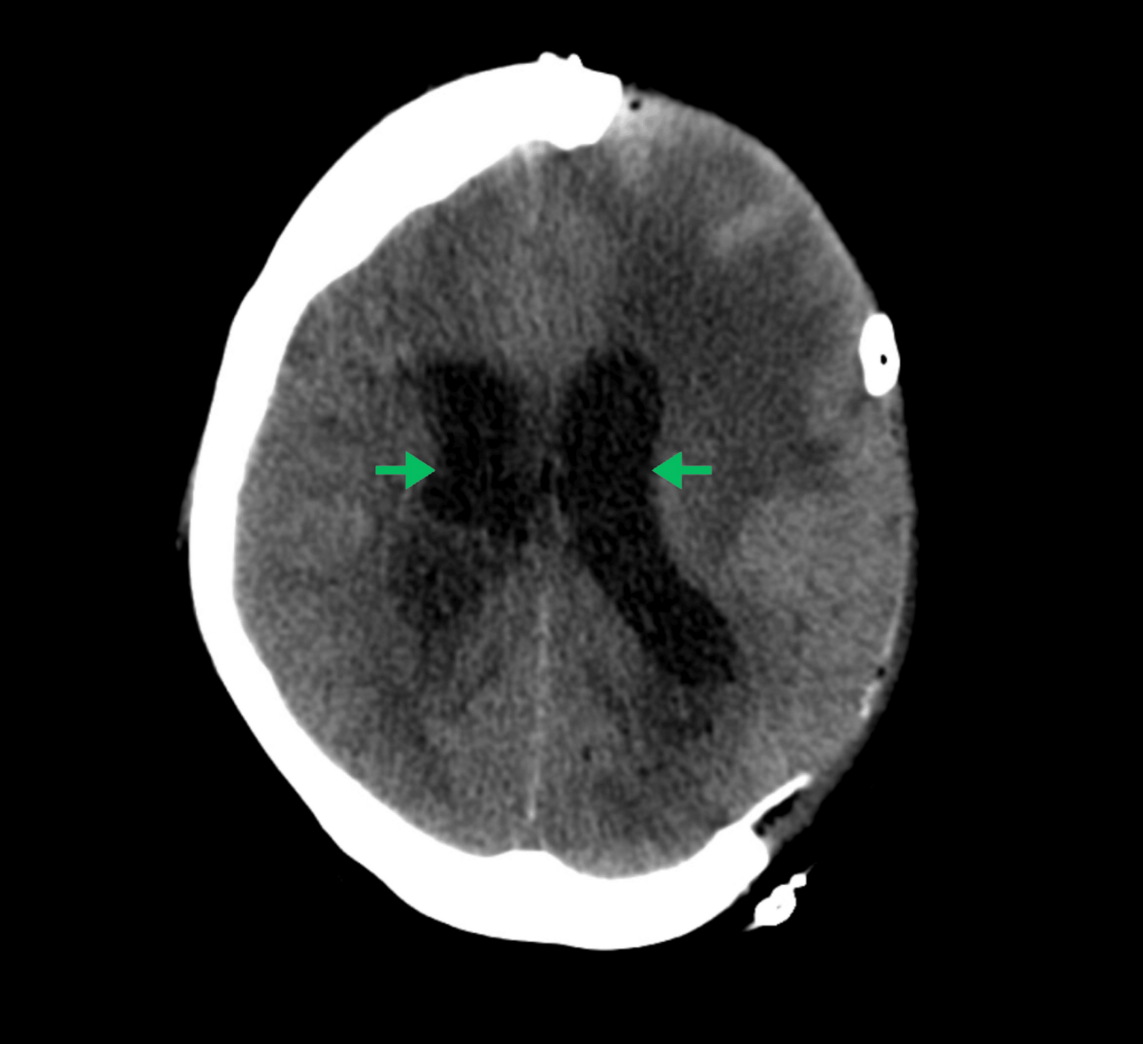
Computed tomography (CT) of the head without contrast demonstrating moderate dilatation of the lateral ventricles. Green arrows: Bilateral dilatation of the lateral ventricles.
